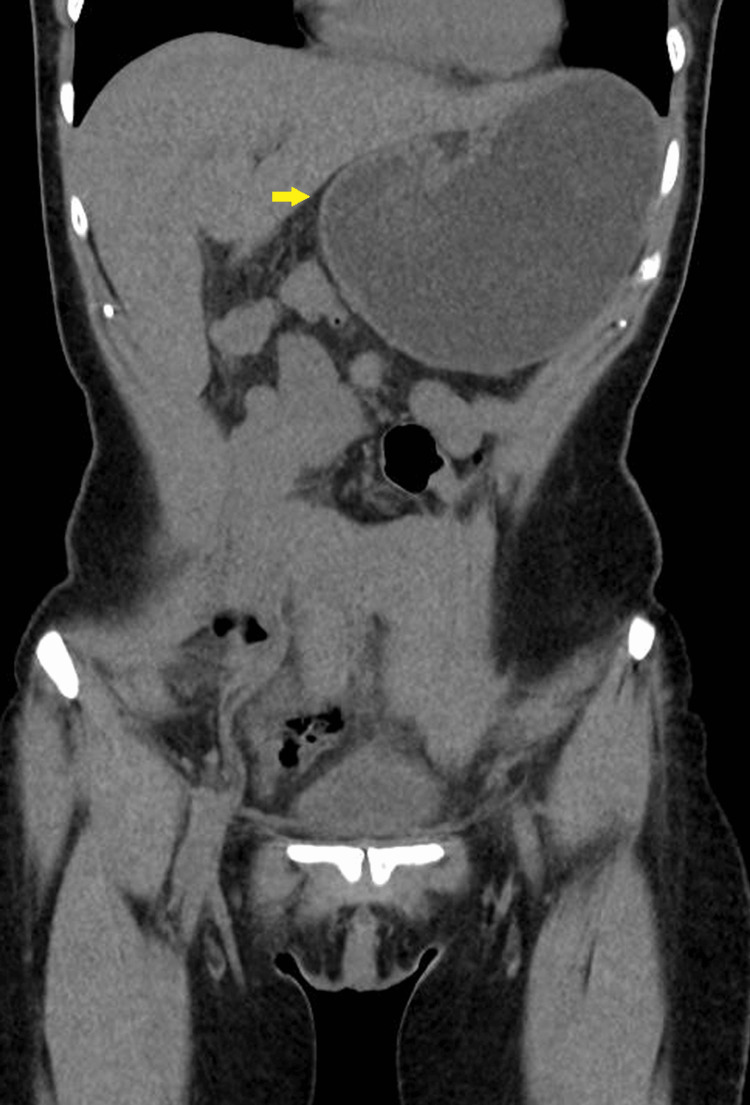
Coronal view of the CT scan of the abdomen and pelvis demonstrating gastric volvulus: the arrow shows the abnormal superior-anterior position of the pylorus CT: computed tomography
